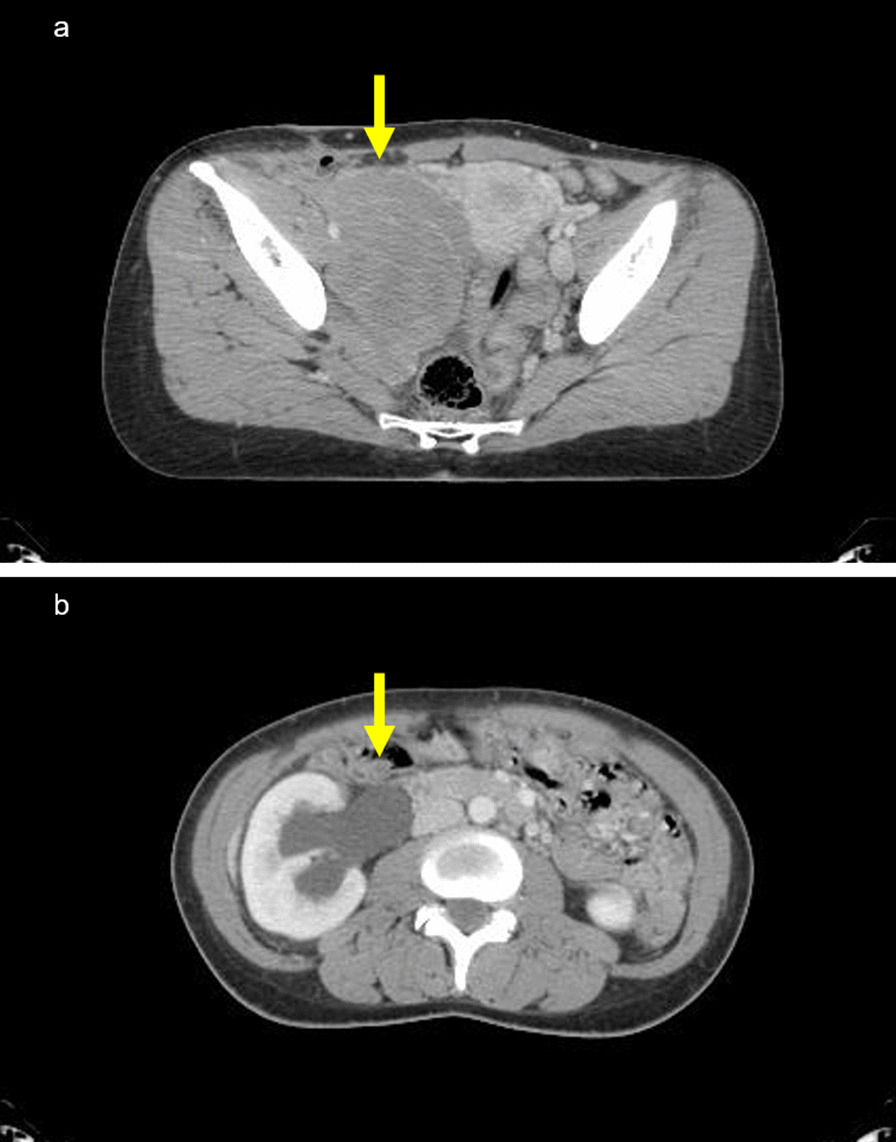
a Axial enhanced computed tomography scan image of the pelvis. The lesion is located close to the iliac artery, uterus, and rectum (yellow arrow). b Axial enhanced computed tomography scan image of the abdomen revealing hydronephrosis of the right kidney. The right kidney and renal pelvis are enlarged. The right renal parenchyma is mildly thin (yellow arrow)
